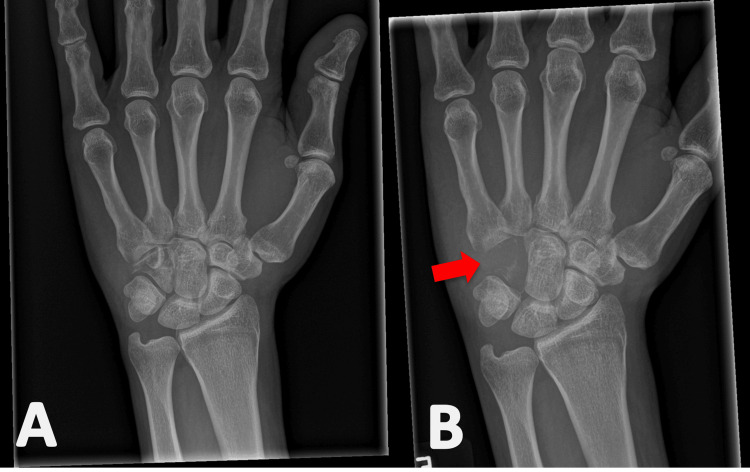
Left wrist posteroanterior radiograph from first presentation to the emergency department showing a normal wrist (A) and eight weeks following the onset of symptoms (B). Profound lytic destruction of the hamate is shown by the arrow.
